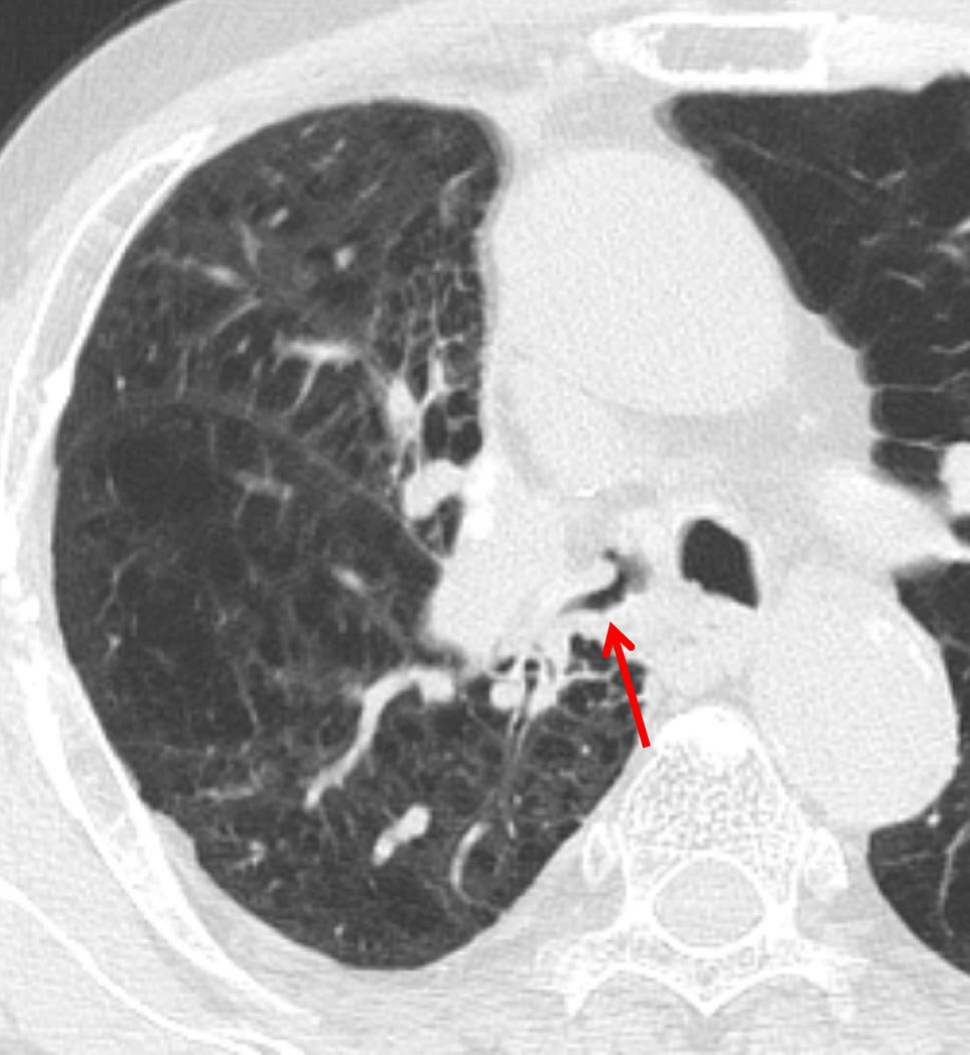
Bronchial anastomotic stricture. Four months after a right upper sleeve lobectomy for lung cancer, CT in the lung window demonstrates marked narrowing at the bronchial anastomotic site
